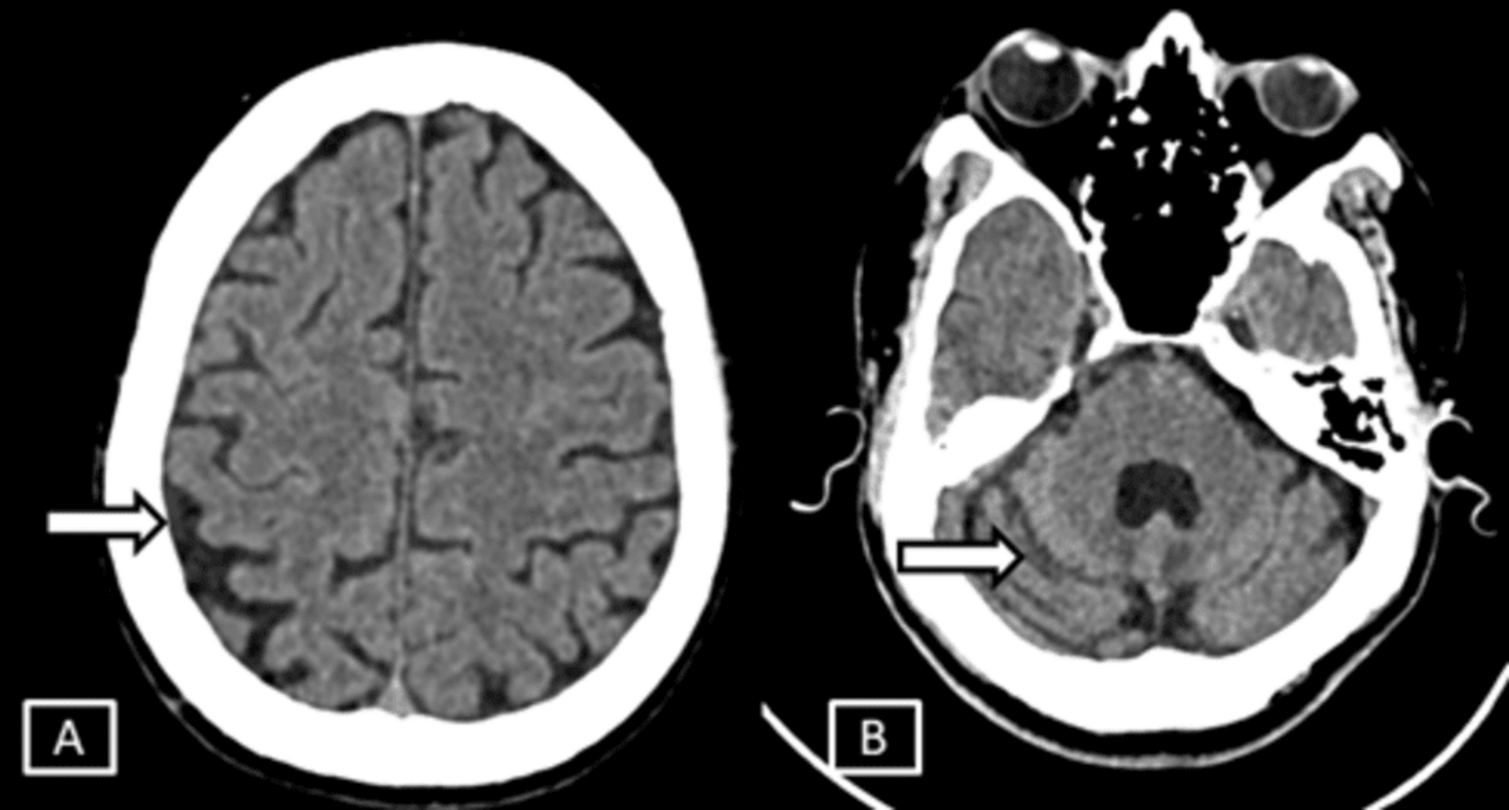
Case 2 CT axial images (A, B) of brain. Images show no significant abnormality except for age-related mild neuroparenchymal atrophic changes in the form of prominent extra-axial sulcal spaces and cerebellar foliae.
